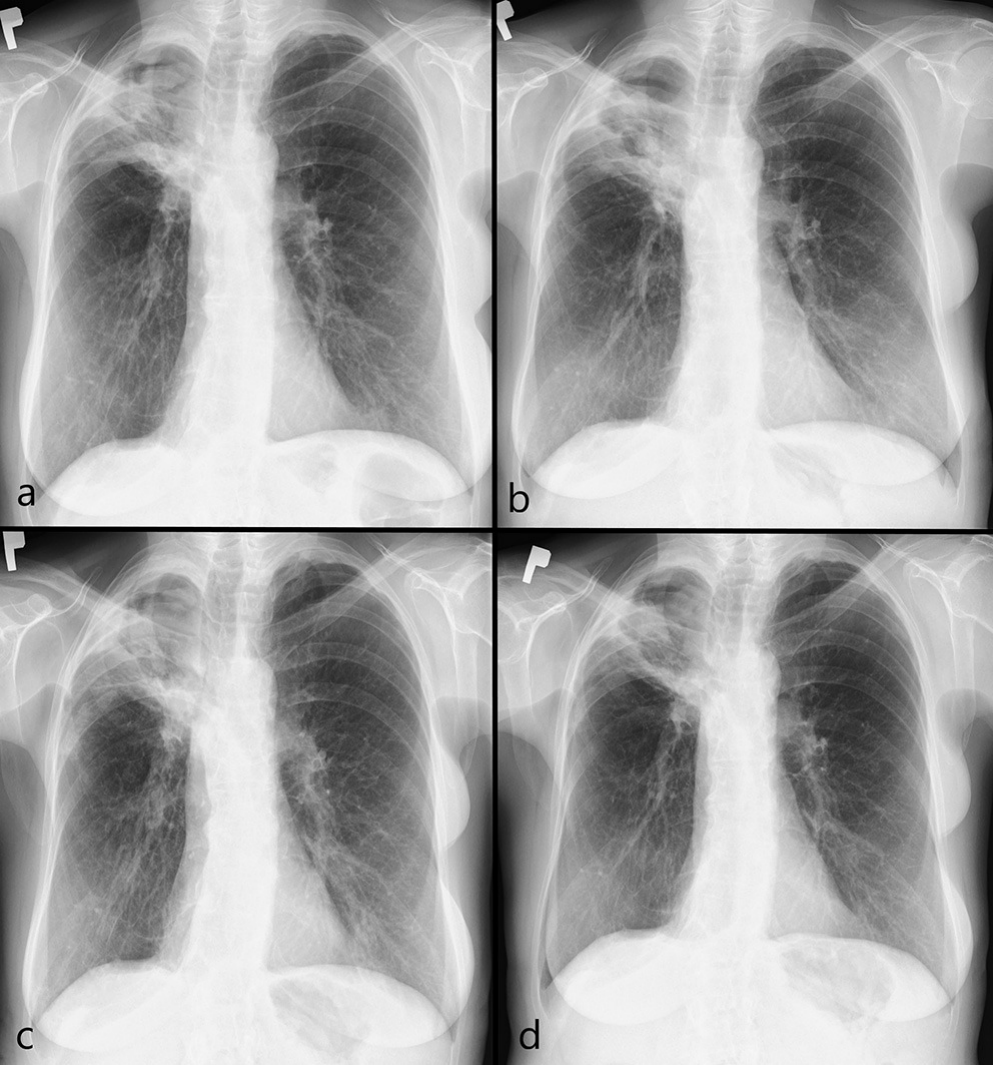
Controlled chest x-ray during first-line treatment in 2020: (a) at the beginning of the therapy – thick-walled cavity with internal consolidations in apical parts of the right lung; (b) after 2 months – cavity consolidation; (c) after 4 months – further cavity consolidation; (d) after 6 months – image stabilization.
